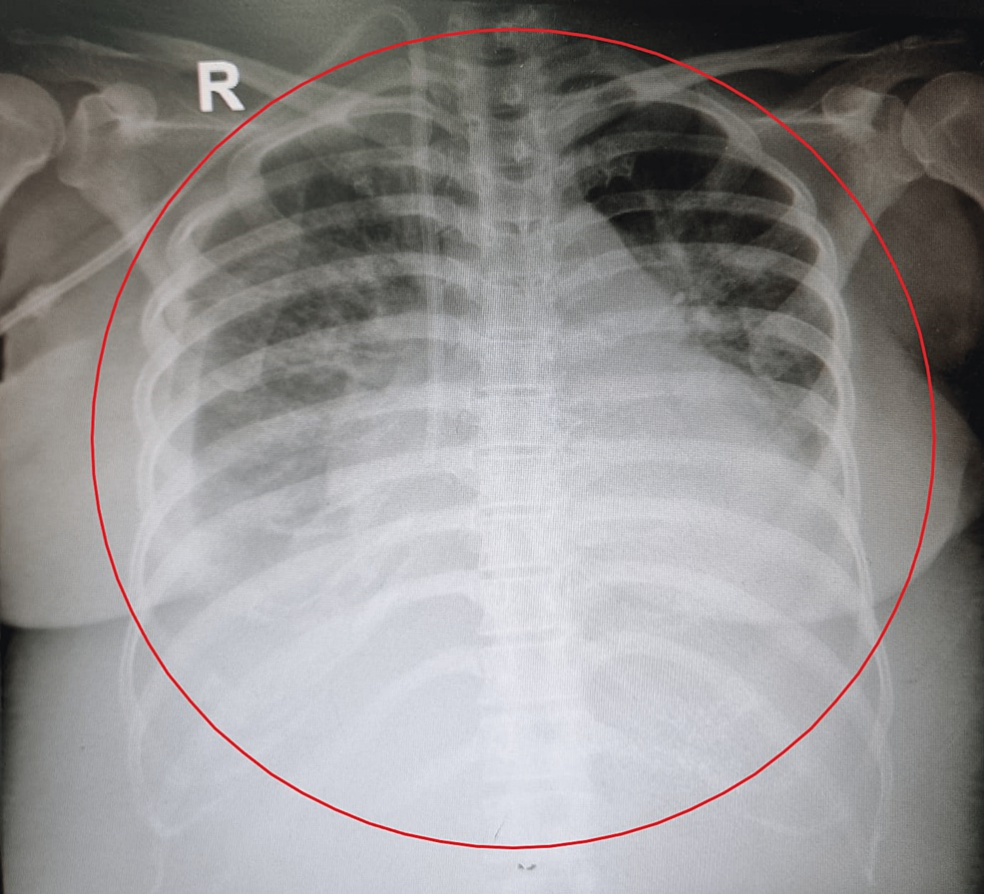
Plain radiography of the patient
